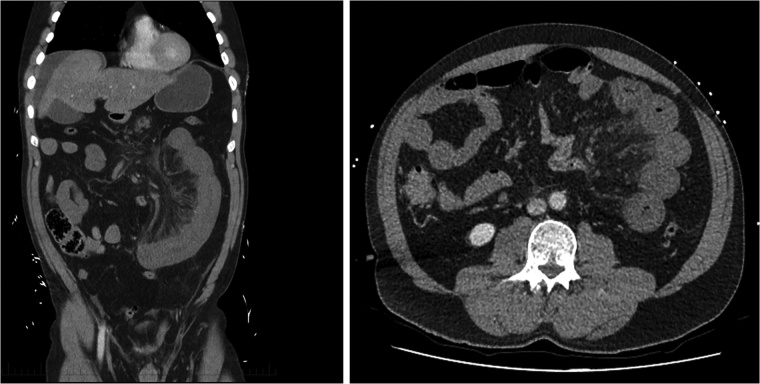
CT demonstrating intestinal ischemia involving a long segment of the jejunum with evidence of bowel wall thickening and fat stranding.
